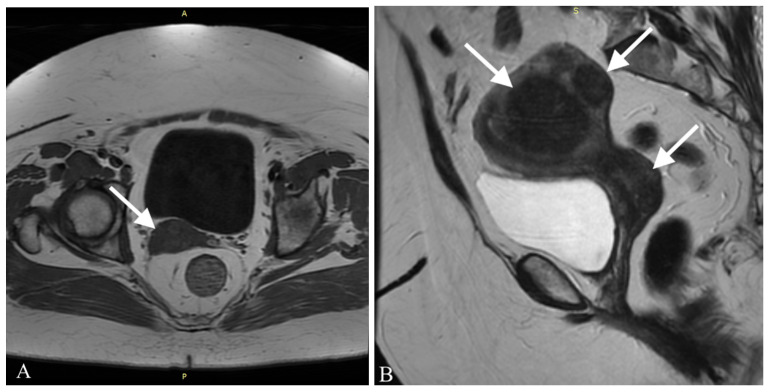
A preoperative MRI of the pelvis in (A) axial and (B) sagittal projection. Both show the presence of myomas in the uterus. Arrows show myomas in the uterus.
